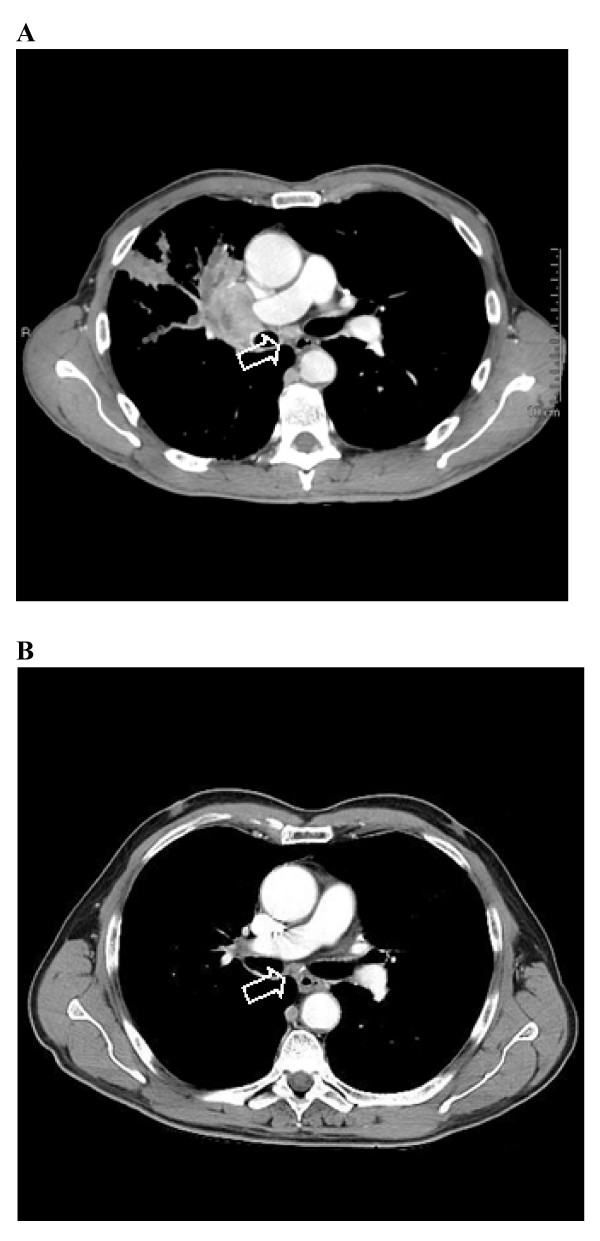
Chest computed tomography scan (A) before treatment of docetaxel (B) 10 months after treatment. The arrows show the mediastinal lymph node metastasis that gradually reduced in size.
